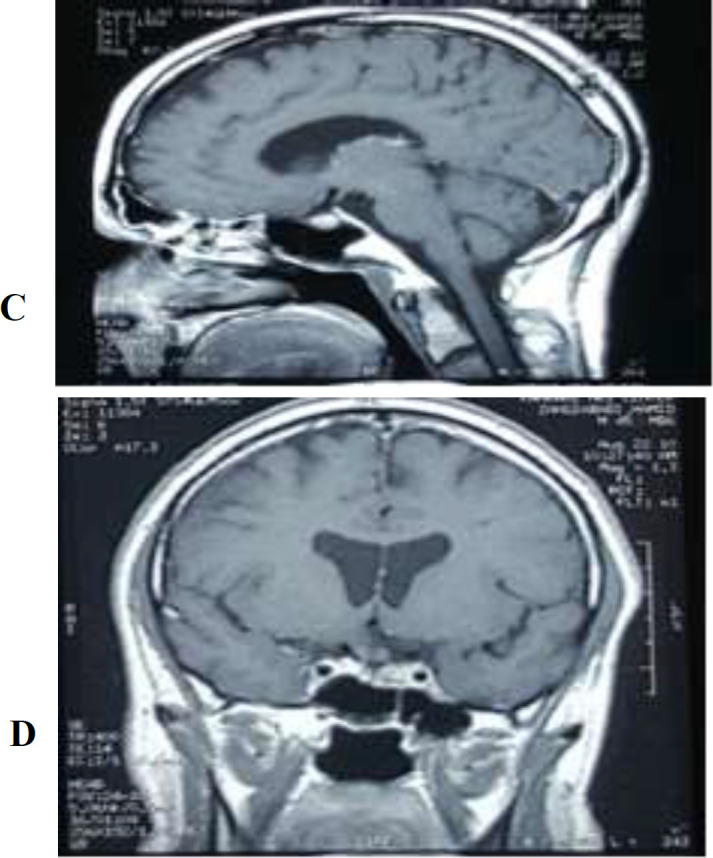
Postoperative T1 imaging (MRI) (sagittal C, coronal D, view) demonstrating a tumor residual in the left cavernous sinus
